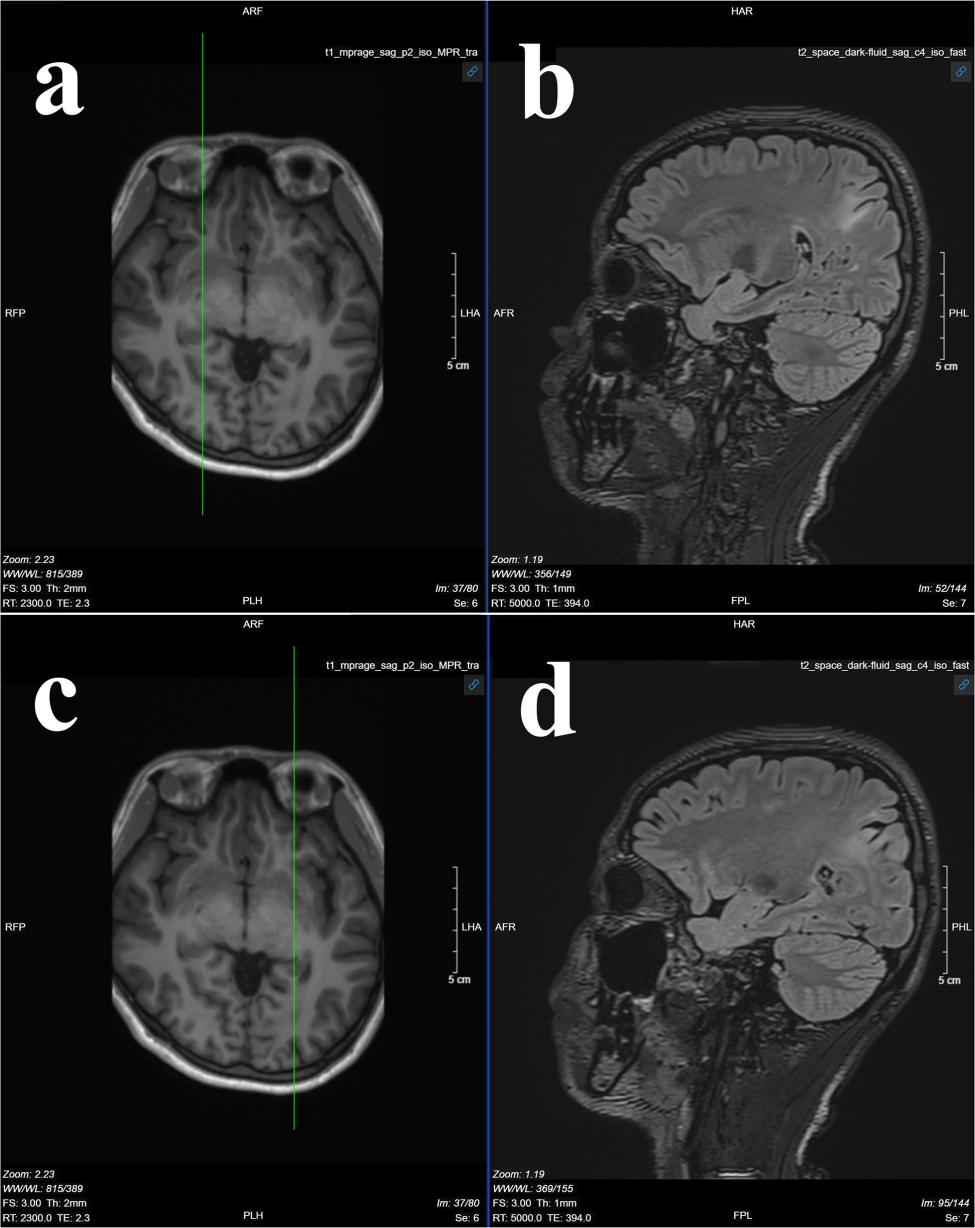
High-resolution hippocampal MRI images showing patchy Flair hyperintensity in the right parietal and left occipital lobes, suggesting the need for contrast-enhanced cranial plain CT scan. Bilateral hippocampal MRI plain scans showed no clear abnormalities. a Transverse image. b Sagittal image, patchy Flair hyperintensity in the right parietal lobe. c Transverse image. d Sagittal image, patchy Flair hyperintensity in the left occipital lobe
